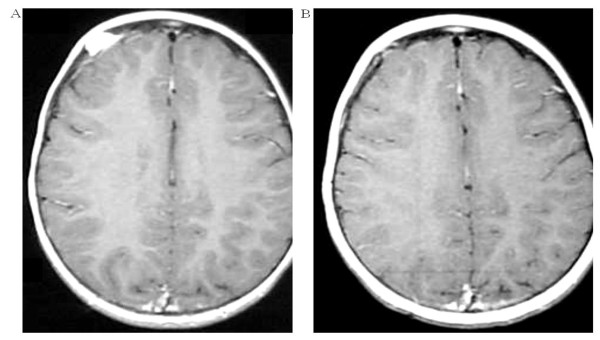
Complete response to vinblastine chemotherapy. Dura mater lesion. Axial T1-weighted magnetic resonance imaging (MRI) with gadolinium before treatment with vinblastine (A) and 8 months after treatment initiation showing a complete response (B).
